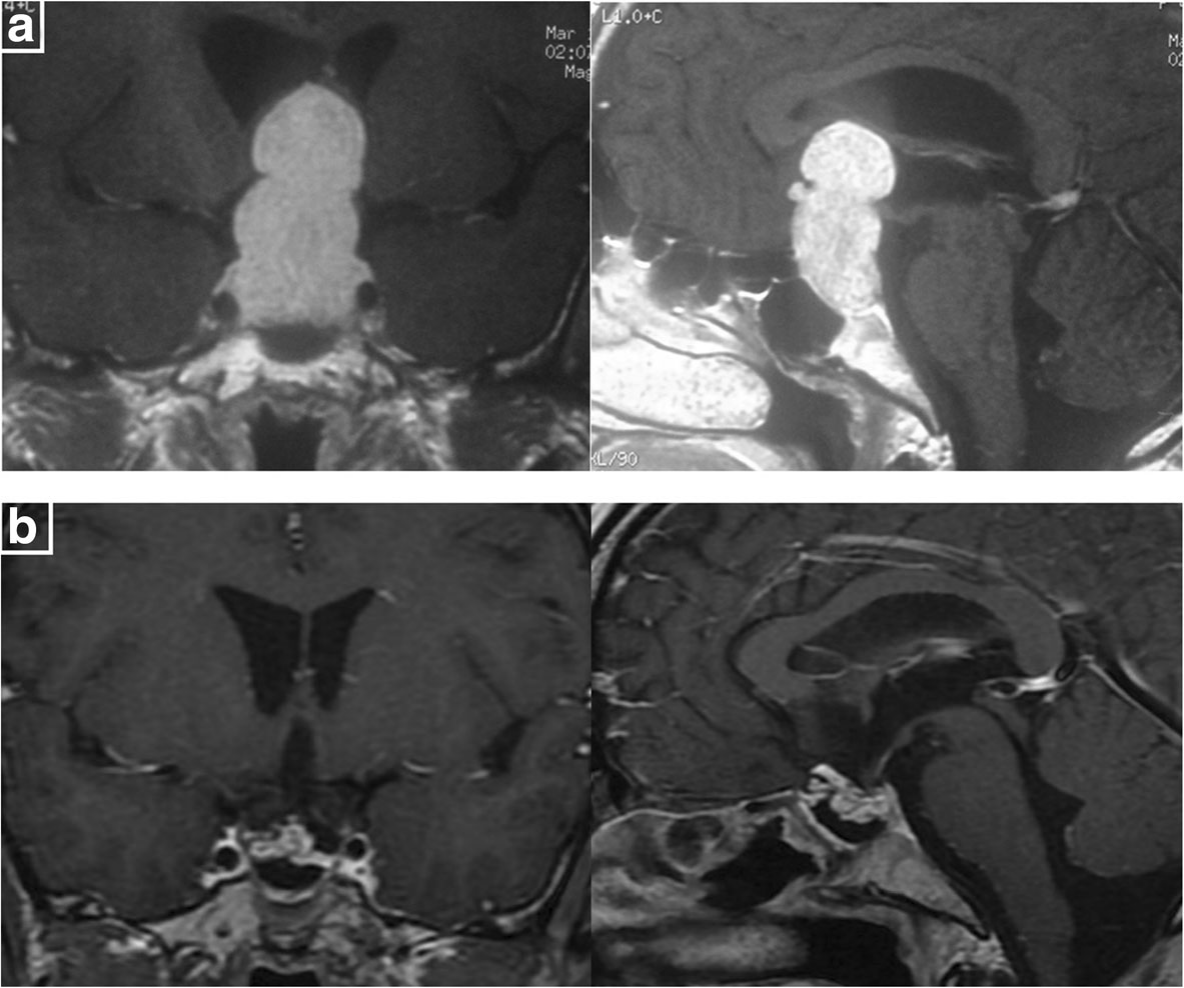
a Preoperative MRI shows giant pituitary adenoma, with a dilation of 5.0 cm, compressing foramen of monro. b Postoperative MRI shows the total resection of tumor
